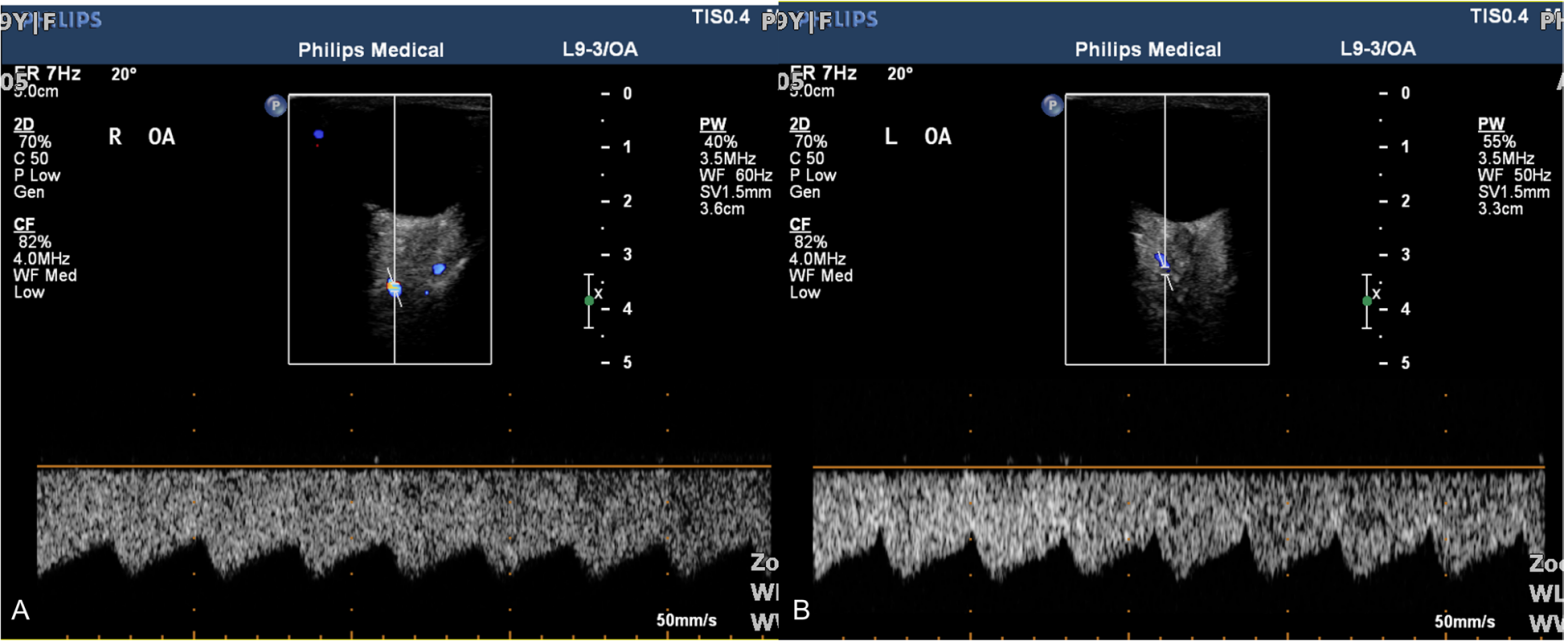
Ultrasonography of the ophthalmic artery. Ultrasonography of the ophthalmic artery showed reverse blood flow in the bilateral ophthalmic arteries
